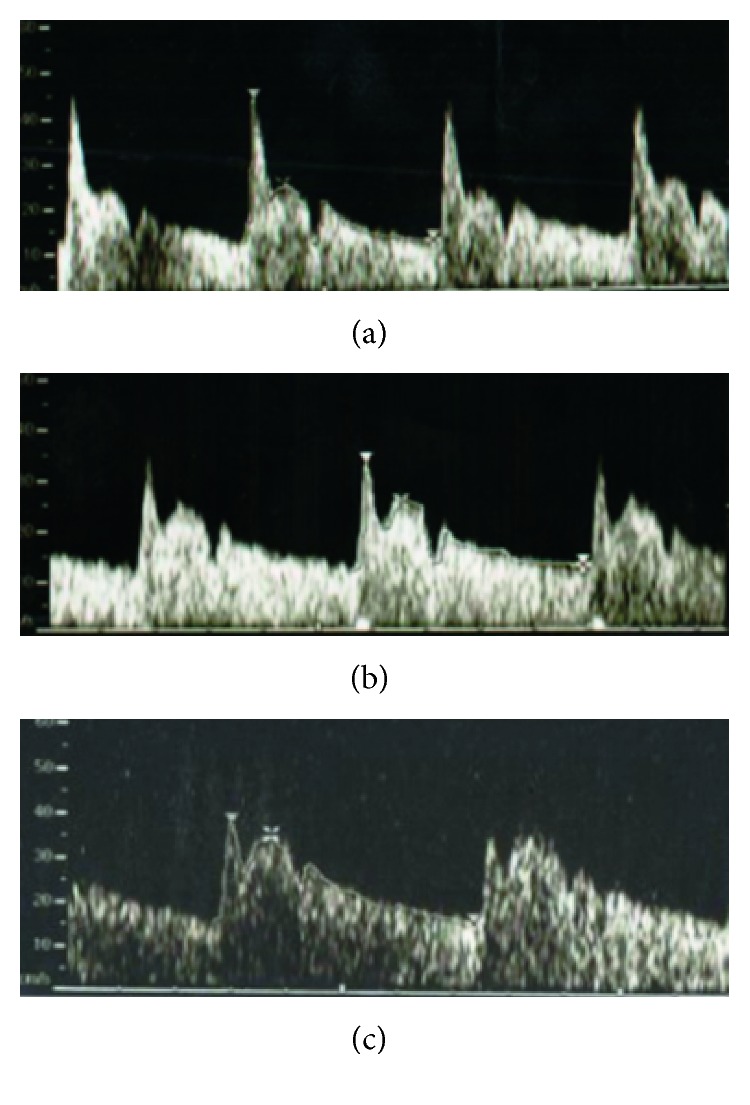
Representation of Doppler velocimetry of ophthalmic artery in a normotensive volunteer (PR = 0.56; P2 = 24.25) (a) and in volunteers with late-onset preeclampsia (LOPE: PR = 0.78; P2 = 25.24) (b) and early-onset preeclampsia (EOPE: PR = 0.93; P2 = 35.23) (c). PR is the P2/PSV ratio, and P2 is the second peak systolic velocity.
